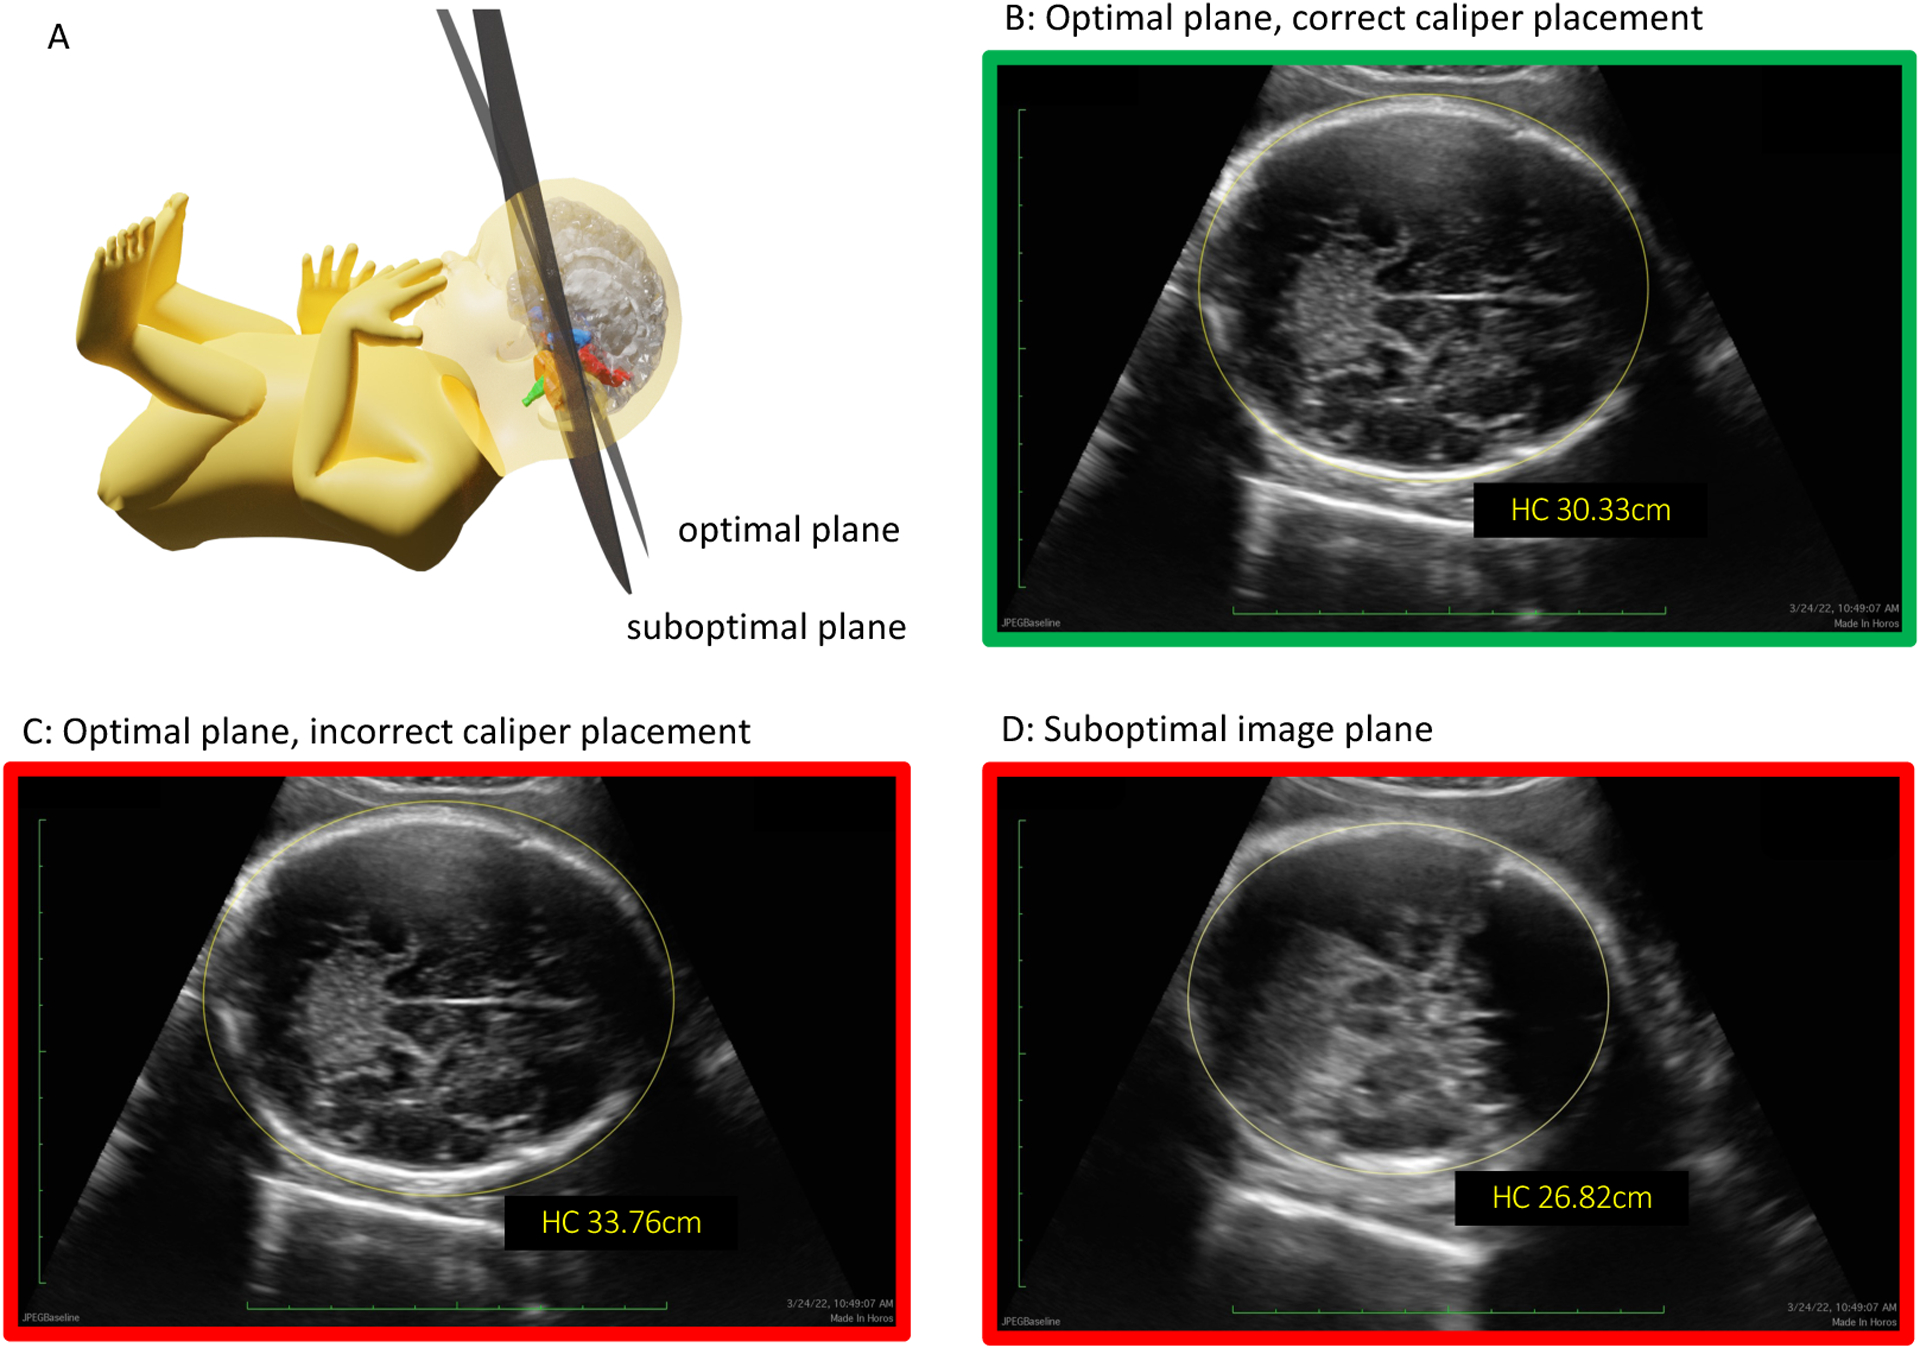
Sources of novice sonographer biometry error Panel A shows optimal and suboptimal image capture. Panel B shows optimal image for measuring head circumference with correct caliper placement. Biometry errors can result from either incorrect caliper placement (Panel C) or from capturing an imperfect plane that distorts the structure of interest (Panel D).
